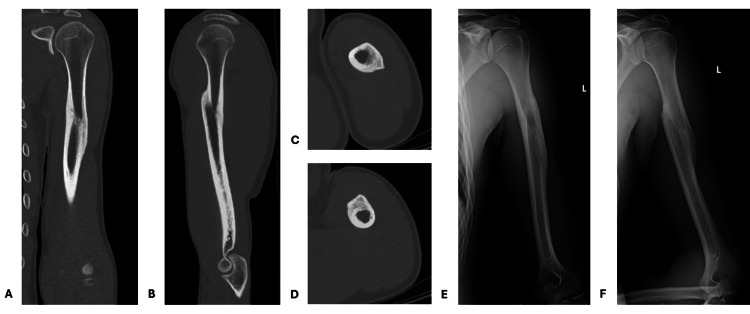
Select coronal (A), sagittal (B), and axial (C, D) cuts of computed tomography scan 7.5 months post-injury (6/7/2024). Anteroposterior (E) and lateral (F) radiographs one year post-injury (10/23/2024)
